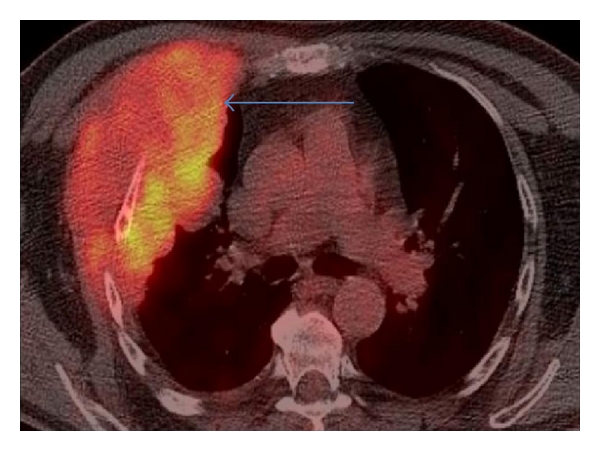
Axial fused PET/CT thorax at the level of the pulmonary bifurcation: massive right-sided chest wall plasmacytoma (arrow).
